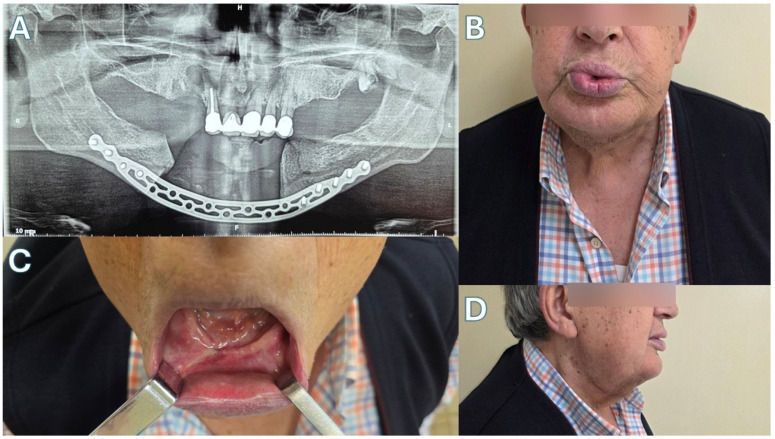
Case 2. (A) Post-op panoramic X-ray. (B–D) Extraoral and intraoral views of the patient, 1 month post-op.
